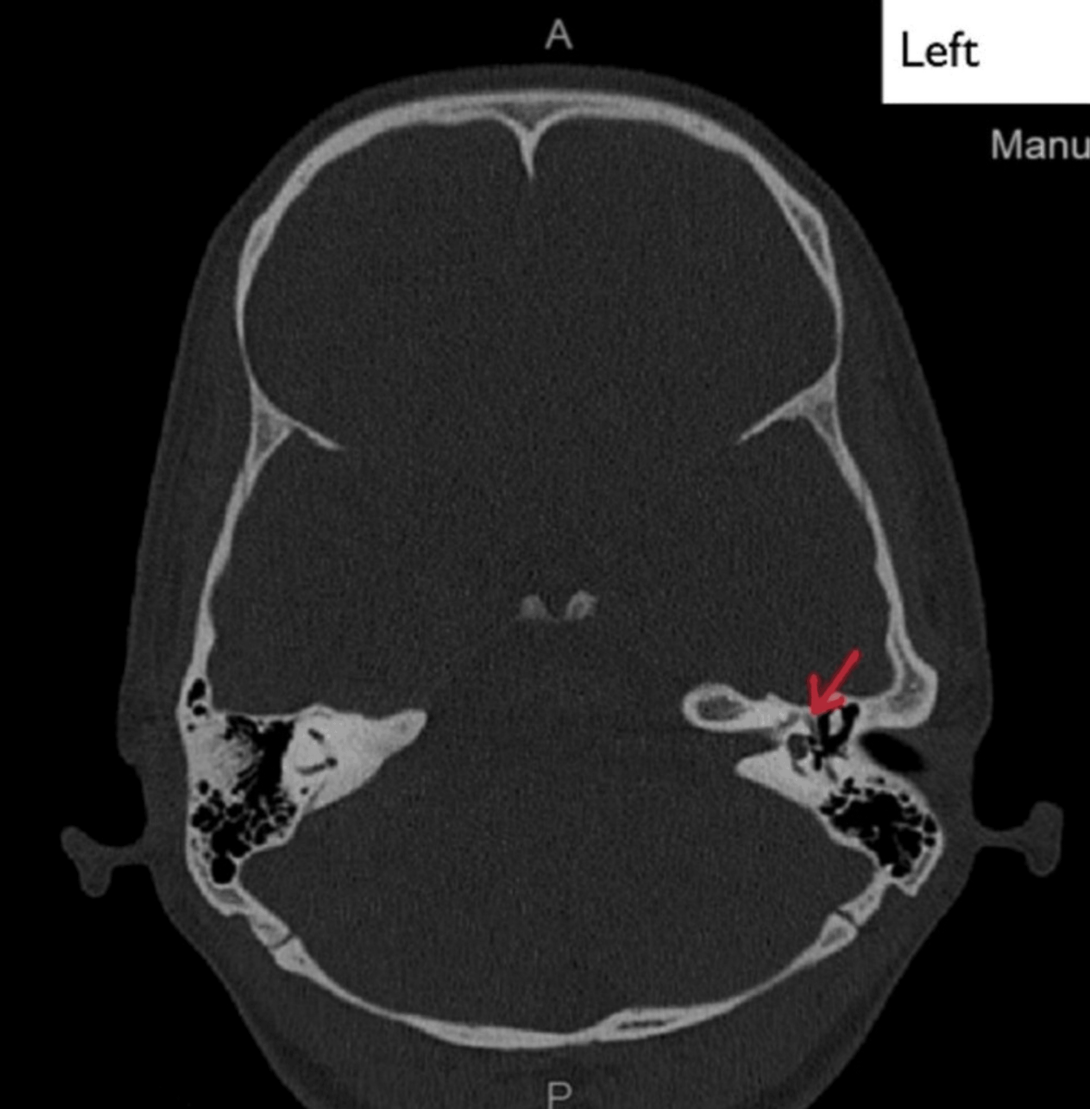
Coronal section of high-resolution computed tomography of the left temporal bone. The red arrow points to the oval window niche and facial nerve.
